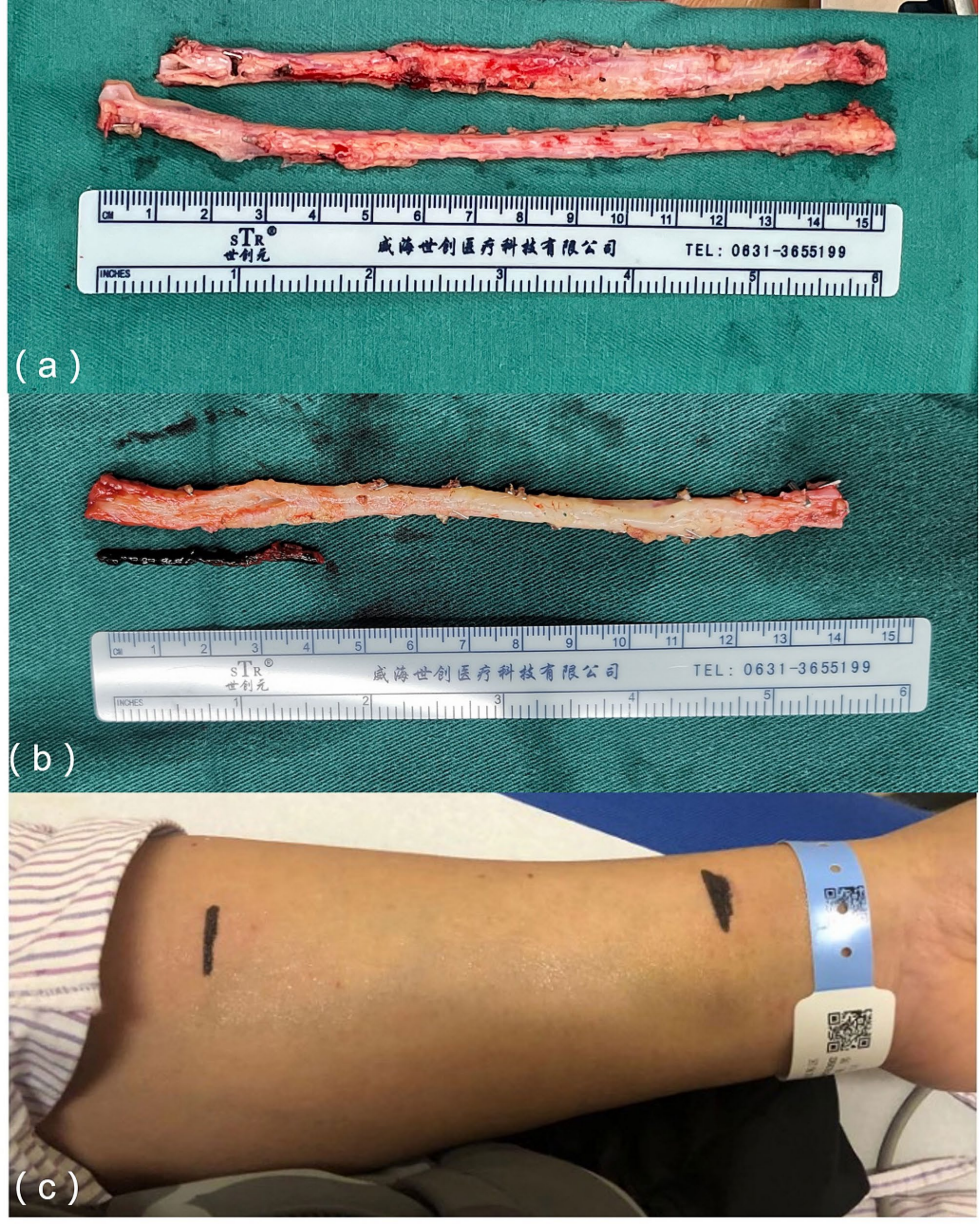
(a) The radial artery was harvested using the “NO-TOUCH” technique, and the target blood vessel was selected based on the measured length; (B) The intima of the remaining bypass grafting vessel remained intact after the distal thrombus was removed and flushed from the distal end of the radial artery; (C) Preoperative evaluation of the radial artery intima and lumen diameter was conducted via ultrasound and marked on the body surface
